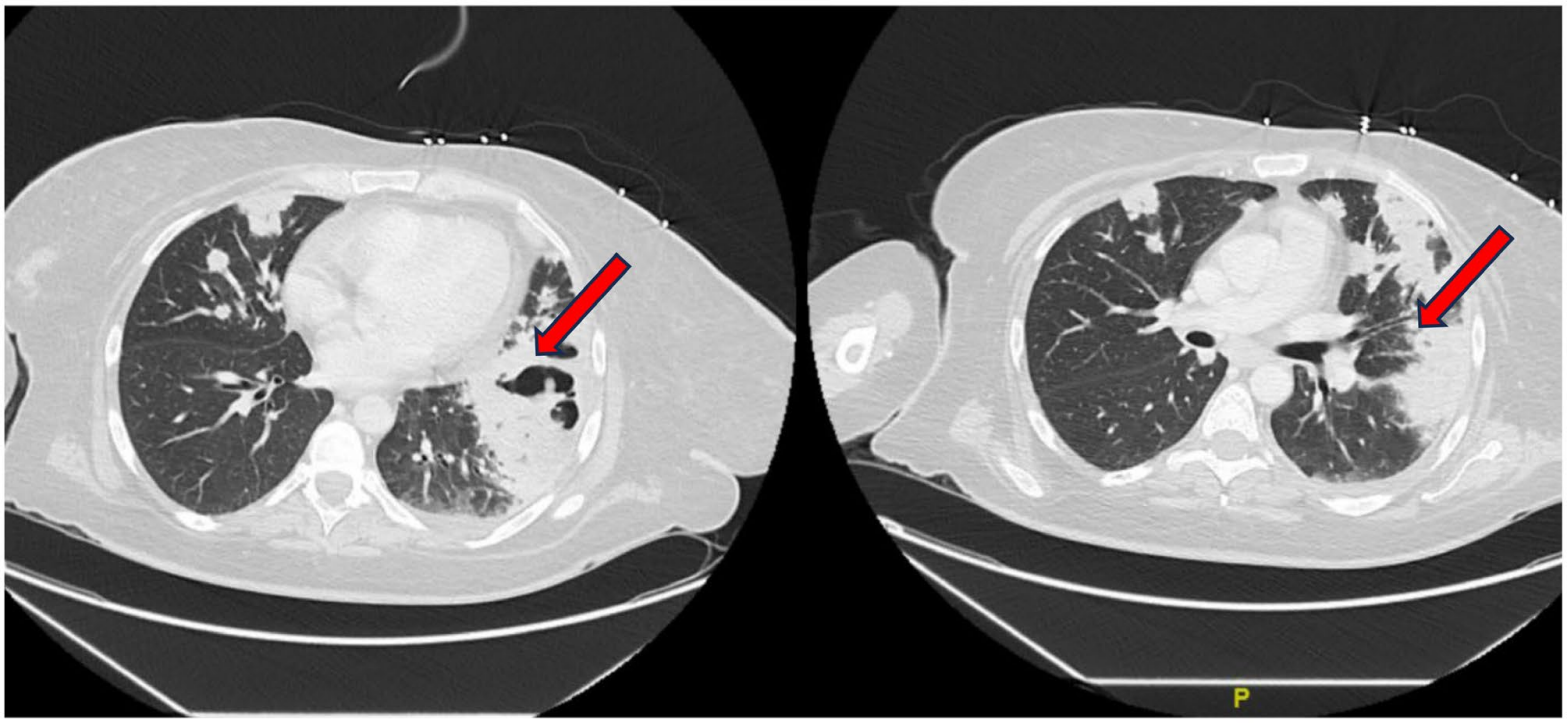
Initial computed tomography (CT) of the chest 07/2019: Extensive bilateral pulmonary consolidation, cavitary and noncavitary consolidative nodules, and suspected peripheral left lung base abscess, some pleural effusion.
